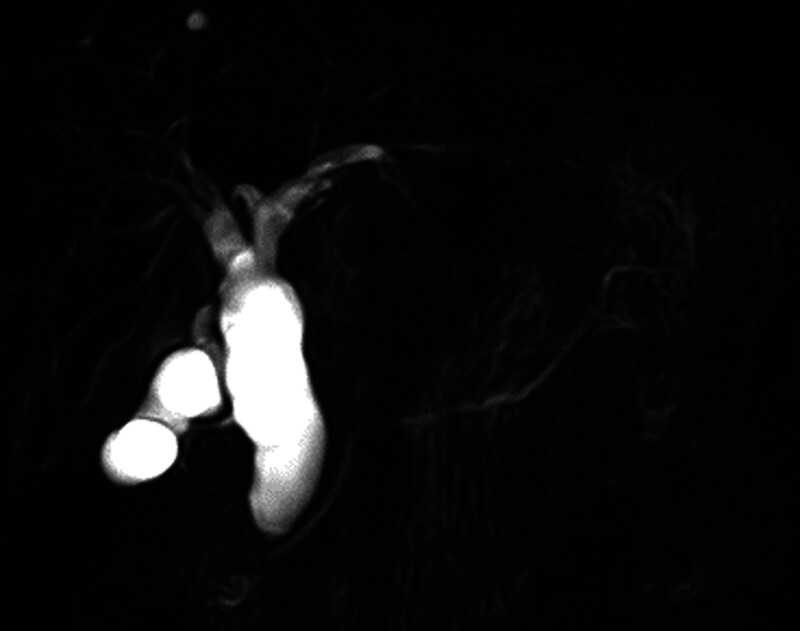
T2-weighted magnetic resonance cholangiopancreatography showing spindle-shaped dilatation of the common bile duct without lymph node swelling around the cystic duct.
